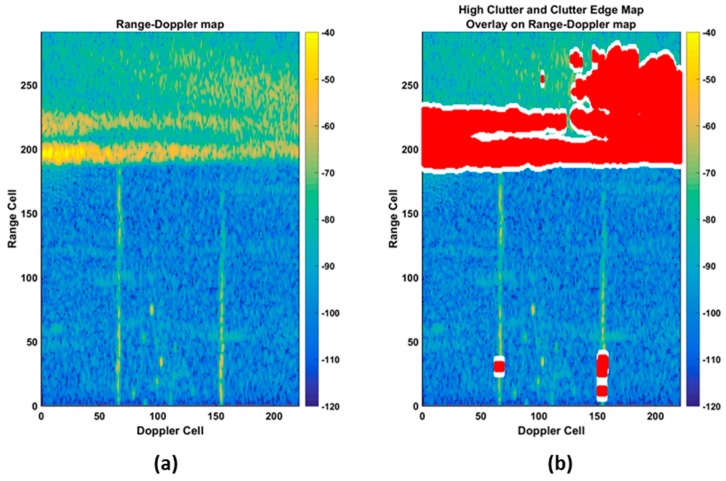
(a) Typical range–Doppler plane, with (b) high-clutter areas, and edges identified with a red and white overlay.
